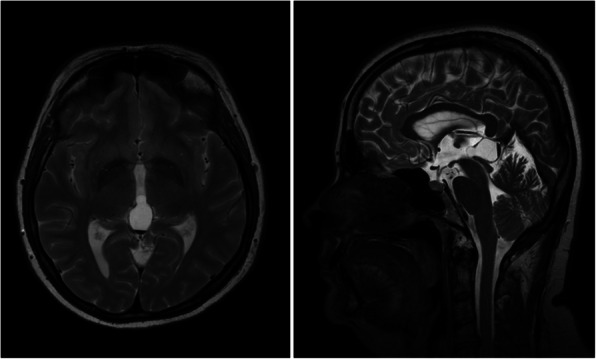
Patient with pineal apoplexy. Left: axial T2-weighted image showing large pineal cyst occupying posterior third ventricle and pineal region. Note the fluid-fluid level showing blood sediment. Right: sagittal T2-weighted image showing the same finding
